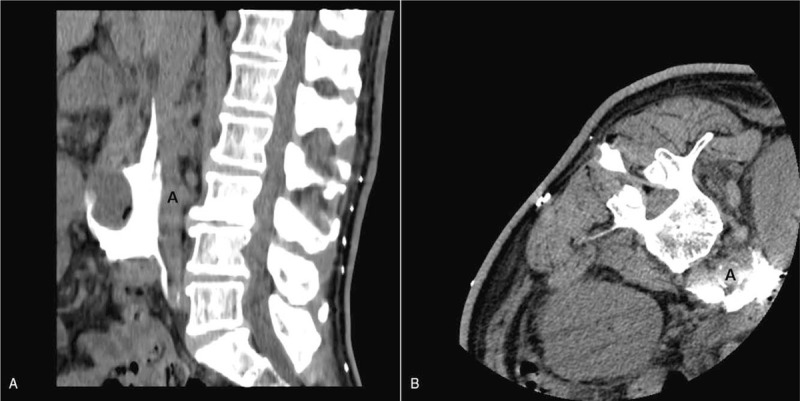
(A) Sagittal computed tomography (CT) image showing that the radiocontrast spreads well from the L2 to the L4 level in the front part of the abdominal aorta. (B) Axial CT image showing that the radiocontrast spreads well over the entire front part of the abdominal aorta. A (aorta).
